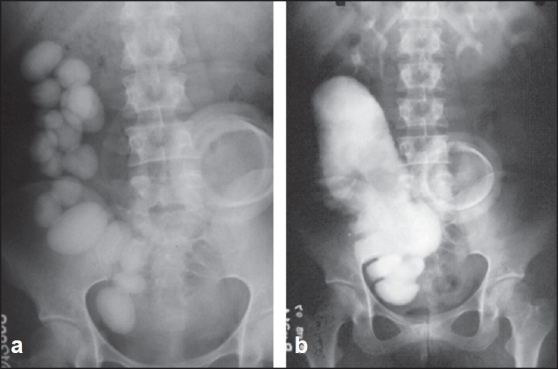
Plain X-ray abdomen (a) shows multiple stones in the right side of the abdomen. Intravenous urogram (b) shows well-preserved kidneys with large reservoir and stones. Circular shadow of colostomy device is also seen
